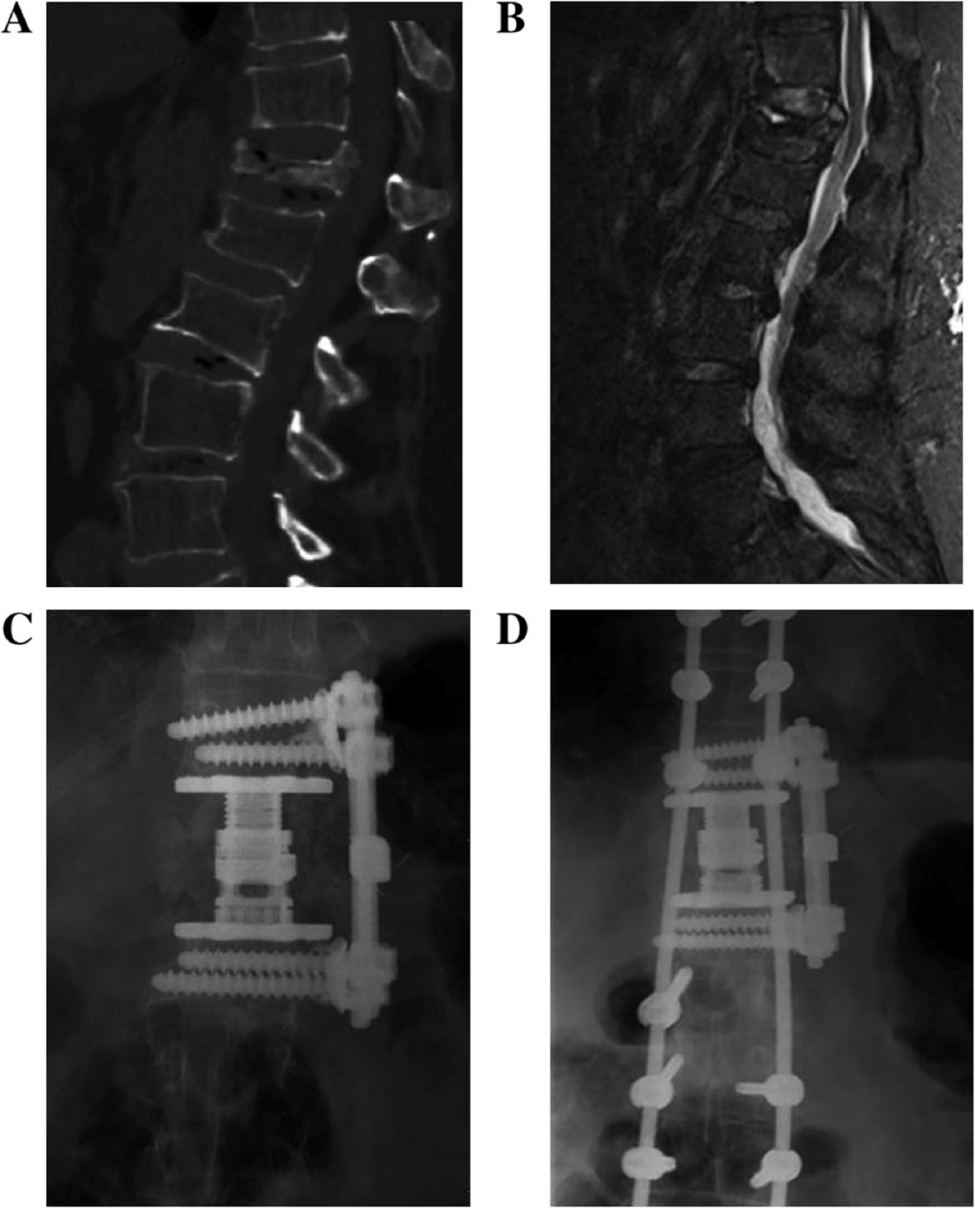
A 58-year-old woman sustained a fall from ground level. (A) lateral x-ray shows burst fracture of T12. (B) MRI shows the burst fracture and canal compromise. (C) AP x-ray show good apposition of the rectangular footplates and the adjacent endplates. One week later the patient was experiencing disabling back pain upon mobilization in thoracolumbar orthosis. Posterior minimally invasive pedicle screws were placed. (D) AP x-ray shows stable and satisfactory spinal alignment.
